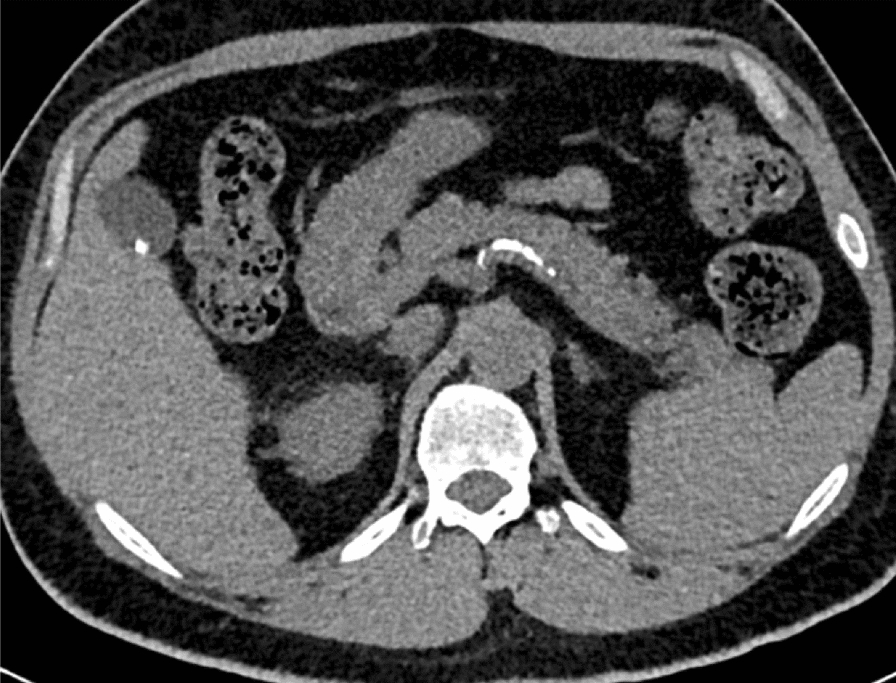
Multi-detector computed tomography without contrast. Calcifications in the splenic artery’s wall in the pancreas parenchyma
